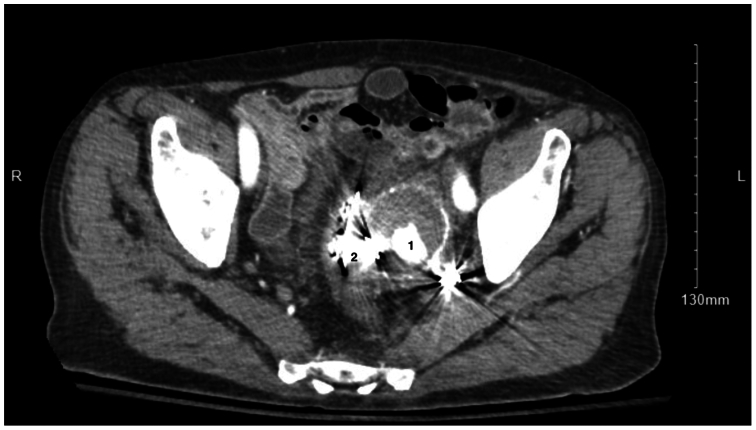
A preoperative computed tomography (CT) axial cut of the pelvis on the day of index surgery showing a patent left internal iliac artery (*1*) with surrounding aneurysm as well as contrast extravasation into the distal sigmoid colon (*2*).
